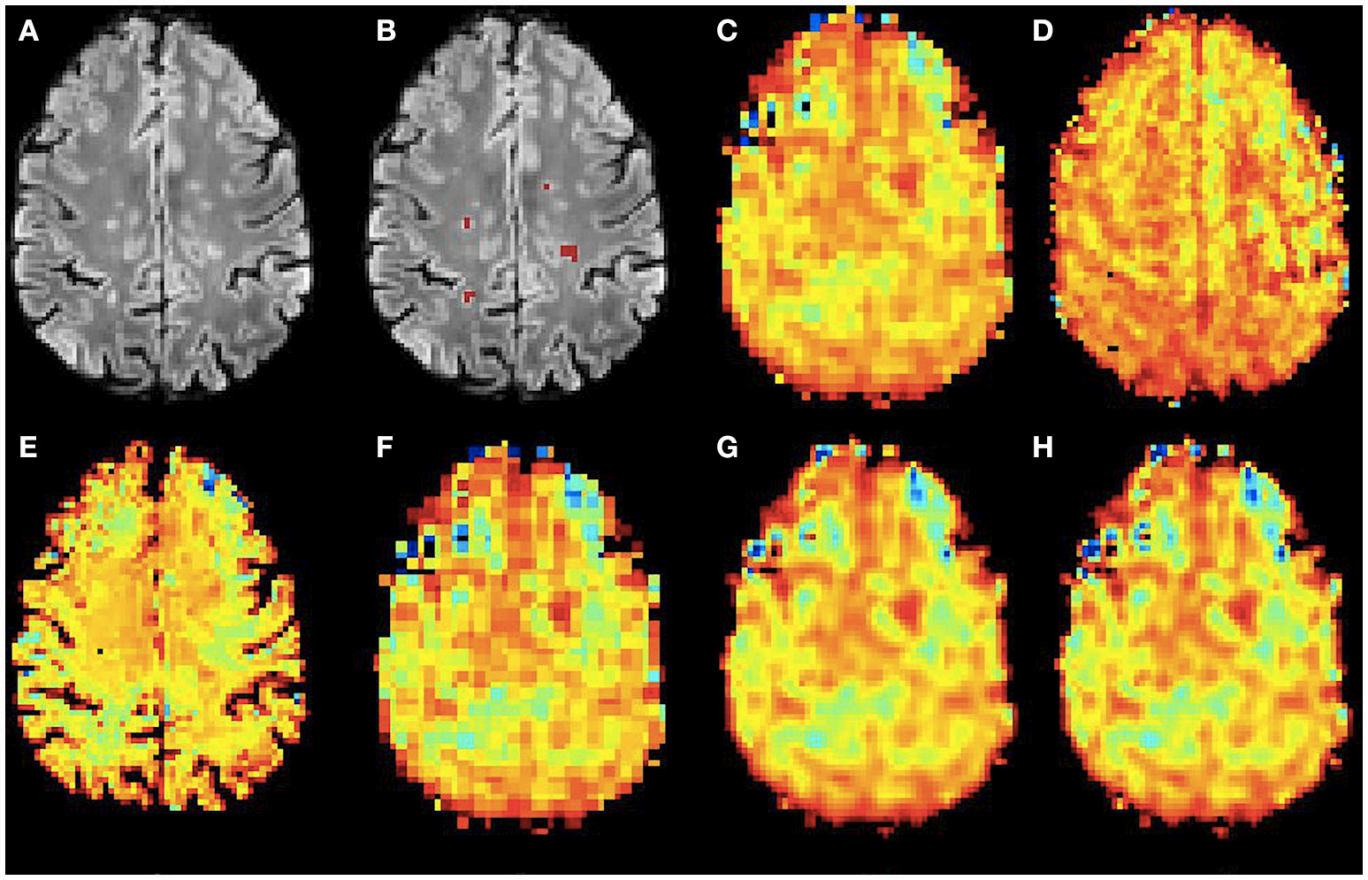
Qualitative accuracy performance of all methods on human dataset acquired *in vivo* for NAA metabolite. (A) Bias corrected FLAIR image followed by (B) overlaid lesion segmentation, (C) the acquired low resolution NAA map; (D–H) the high resolution NAA maps: (D) acquired, (E) PBSR, (F) NN, (G) LIN and (H) BS. The NAA concentration increases from red to blue.
